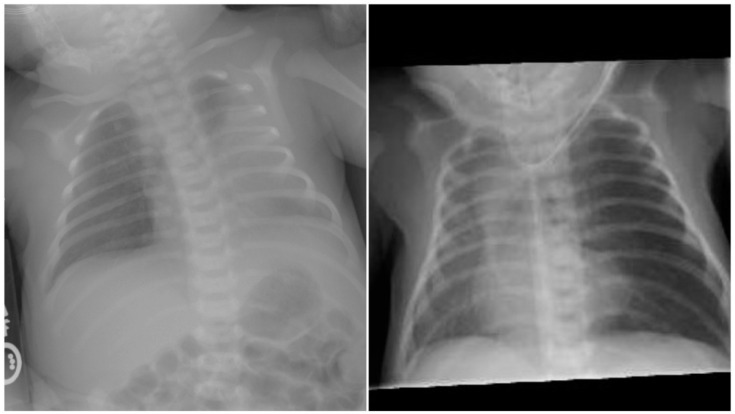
Left panel depicts anterio-posterior chest radiograph on the first day with left-sided opacification. Right panel reveals radiograph at 7 days with hyperinflation of the left and mediastinal shift.
